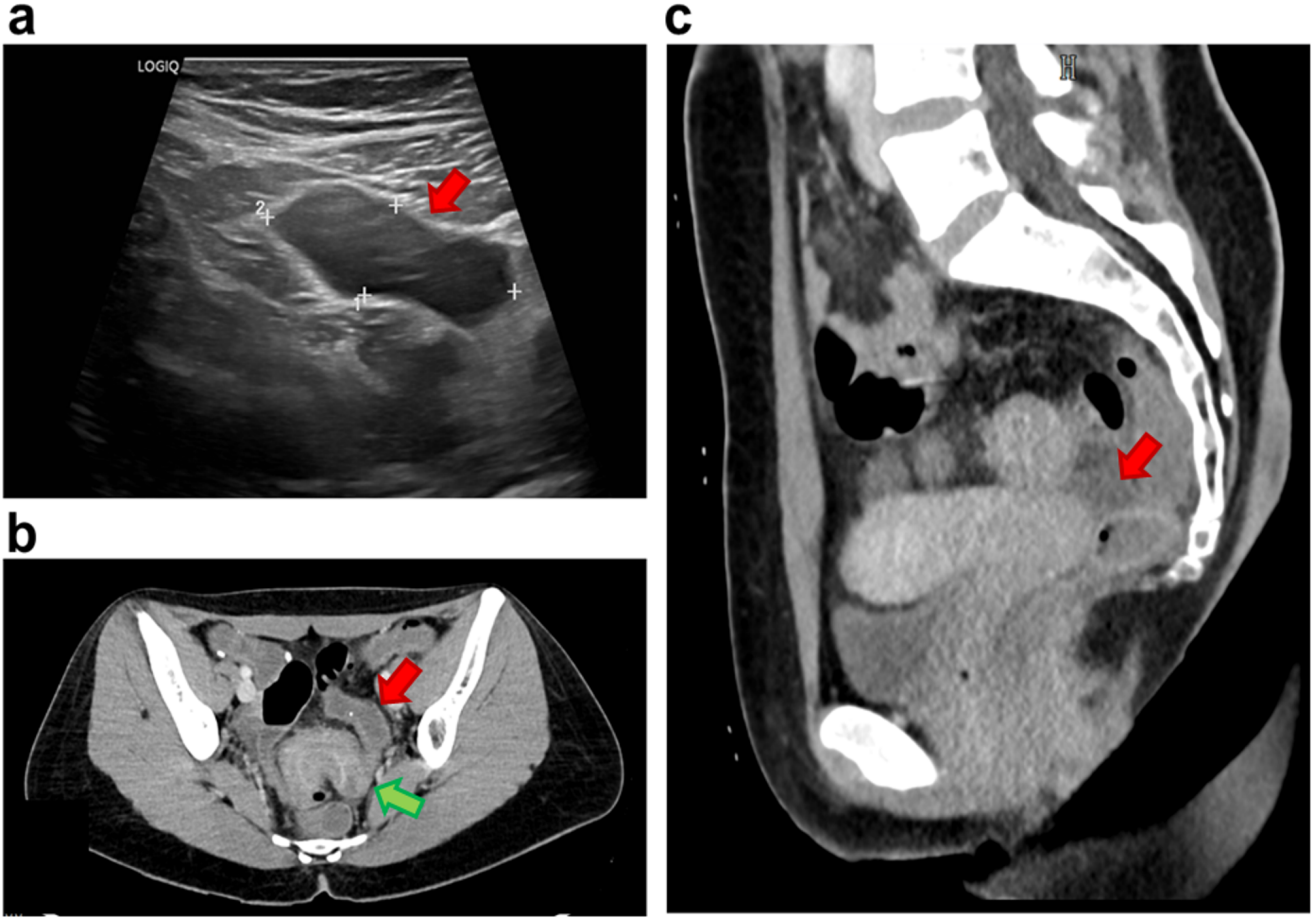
Key radiological findings in Case 1. (a) Ultrasound image showing a 20 × 40 mm anechoic fluid collection in the expected projection of the vermiform appendix (red arrow), which itself is not visualized (red arrow). (b) Contrast-enhanced axial CT scan of the pelvis: The sigmoid colon shows an S-shaped oral segment (red arrow) and a horseshoe-shaped aboral segment (approximately 8–10 cm) (green arrow). The wall of the aboral segment shows significant circumferential thickening, narrowing the lumen. However, the degree of patency cannot be assessed due to the absence of contrast material (the patient vomited before the examination). (c) Contrast-enhanced sagittal CT scan of the pelvis: The uterus and ovaries appear normal. Pathologically enlarged lymph nodes are visible in the examined region. A minimal amount of free abdominal fluid is seen around the sigmoid colon (red arrow)
